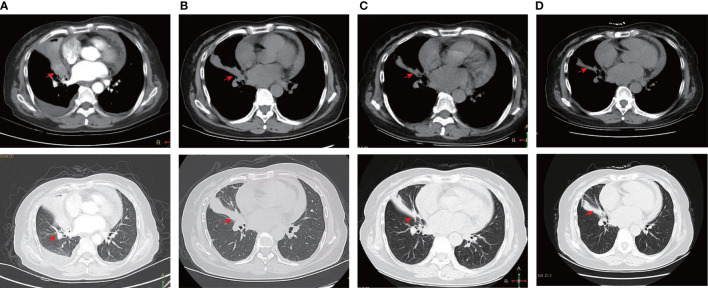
Dynamic imaging of lung lesions at different stages of treatment. (A) CT scan before alectnib treatment (March 1^st,^ 2022); (B) CT scan after two months of alectinib treatment (April 22^th,^ 2022); (C) CT scan after four months of alectinib treatment (June 29^th,^ 2022); (D) CT scan after six months of alectinib treatment (June 29th, 2022); note: the red arrow marks the location of the lesion.
